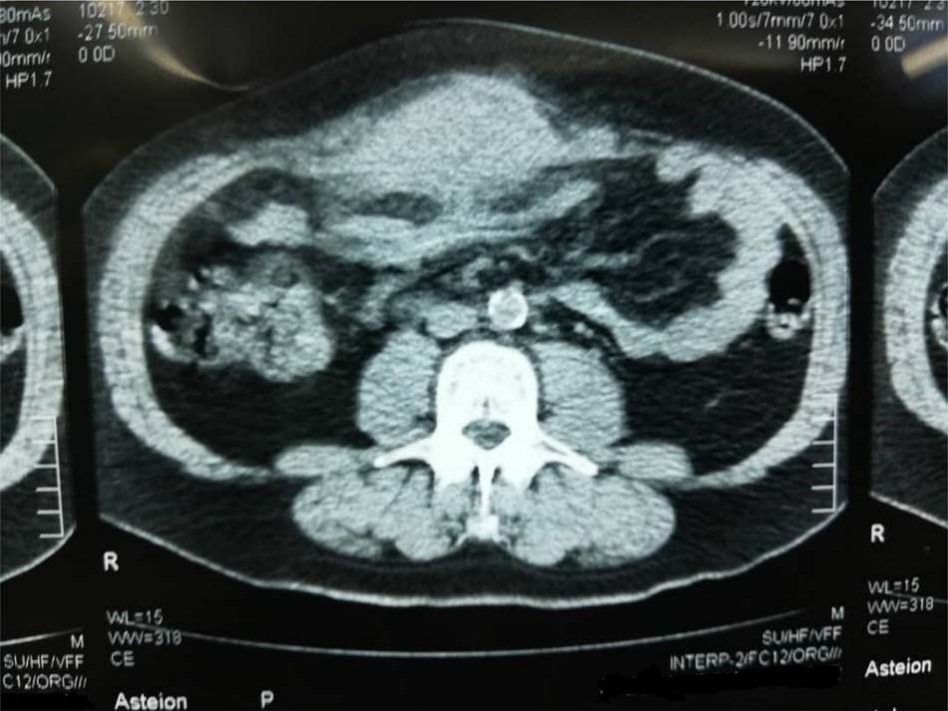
CT scan of the tumor as it shows infiltration to adjacent structures and attachment to the anterior abdominal wall.
